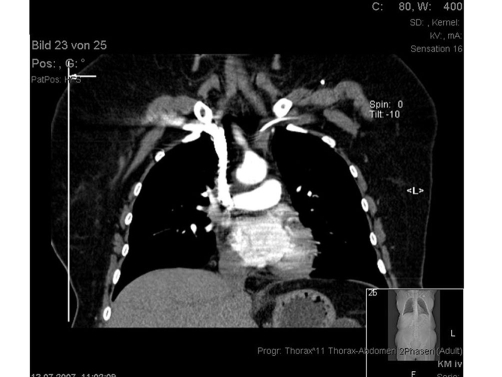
Staging CT-scan preoperatively demonstrating an accurate positioning of the catheter.
